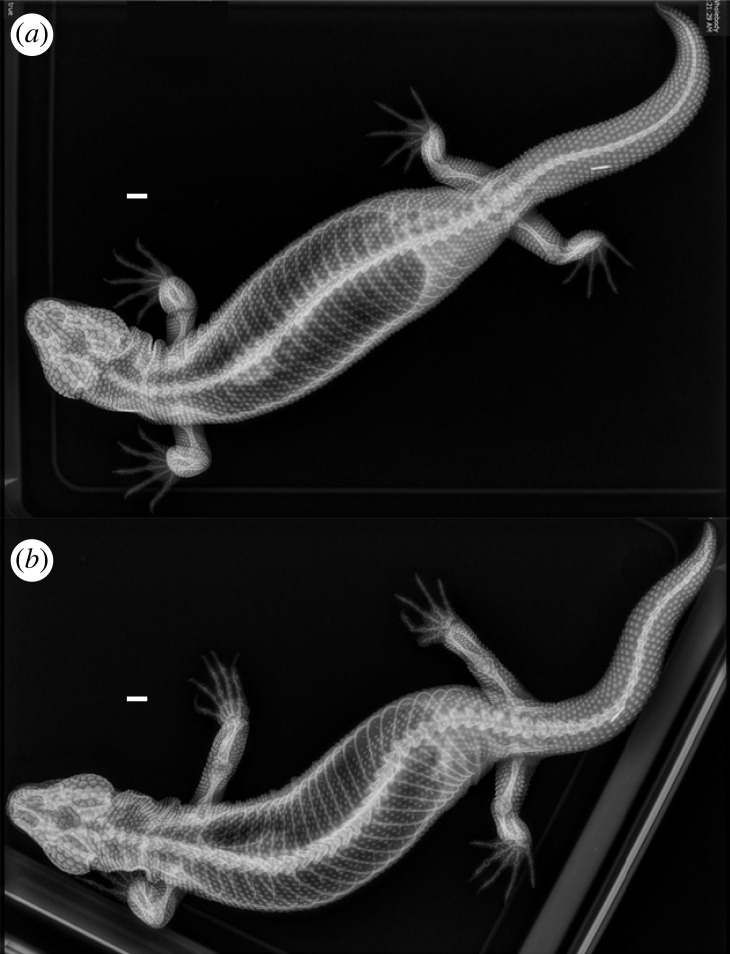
Osteoderms of the Gila monster. Whole body X-rays of (*a*) female (CPP-225) and (*b*) male (CPP-63) *H. suspectum* showing regional variation in osteoderm size and spacing of osteoderms (white dots). The largest and most closely spaced osteoderms are located on the head. Scale bars = 1 cm.
